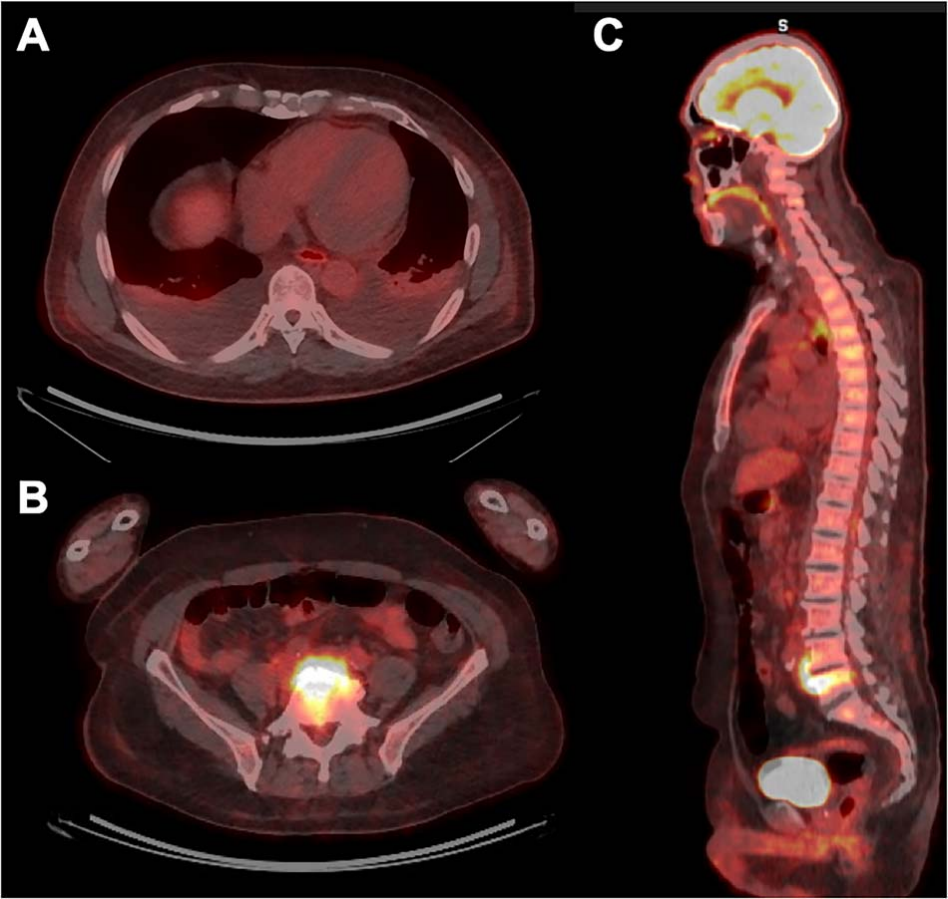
Images of FDG PET/CT. Absence of significant FDG uptake in the whole heart (panel A). Noticeable FDG uptake is observed in L4-5 vertebral bodies (panel B and C).
